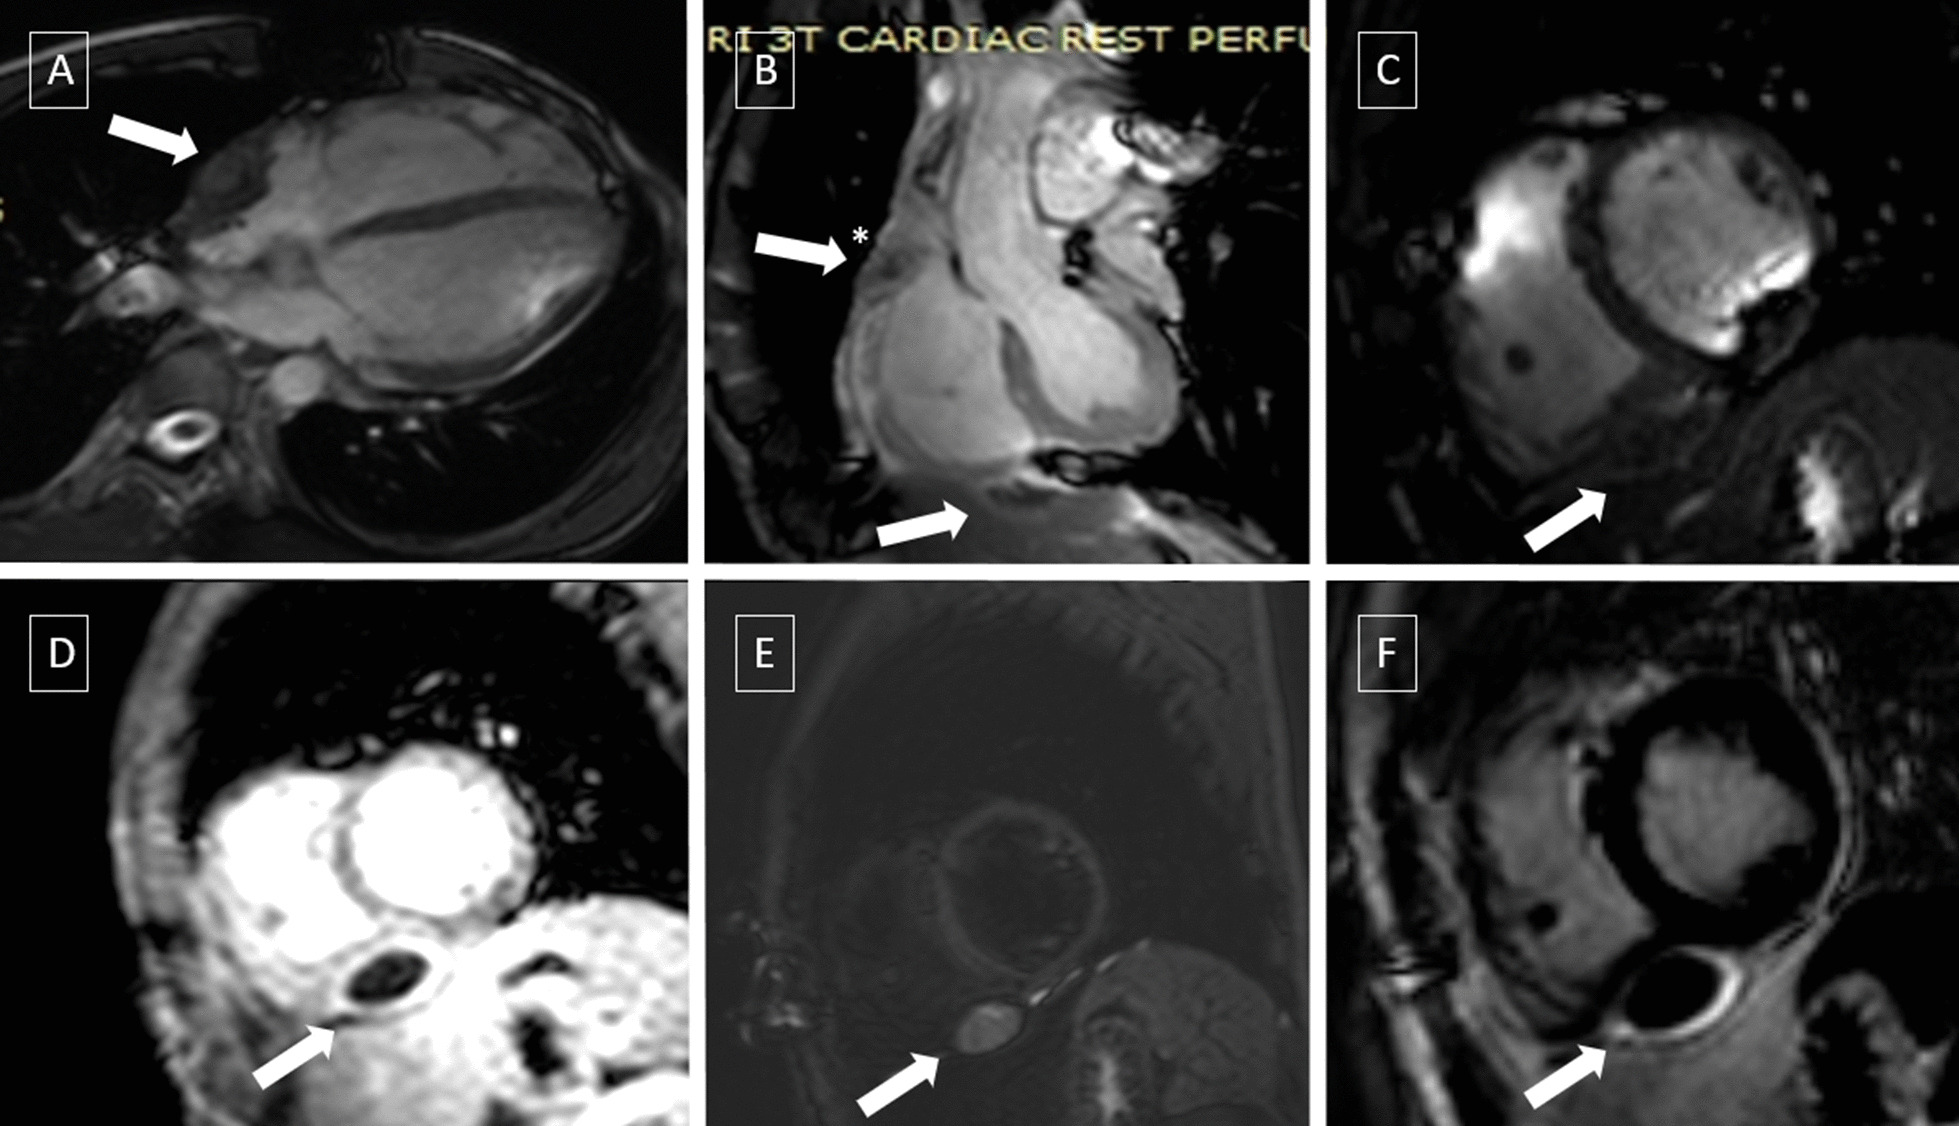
Cardiac MRI. Cardiac MRI was performed with axial sequence white blood, black blood, cine short axis, 4-chamber, 2-chamber, short axis, T2 Short Tau Inversion Recovery (STIR), Look-locker, LGE with Multihance contrast 0.5 mmol/mL, 12 mL. Balance Turbo Field Echo (BTFE) horizontal long axis 4-chambers view aA) showed the mass near the RA was intrapericardial. BTFE ventricular long axis focused-RV view (b) showed compression of superior vena cava (asterisk, *) and intrapericardial mass at RV free wall, demonstrated with BTFE—breath-hold (short-axis view) (c). Low T1 mapping signal on look-locker sequence (d), and heterogeneously high T2 signal ratio (e) evaluated with T2 STIR, with no evidence of gadolinium enhancement (f) with LGE Phase Sensitive Inversion Recovery (PSIR) confirmed the diagnosis of subacute–chronic pericardial hematoma
